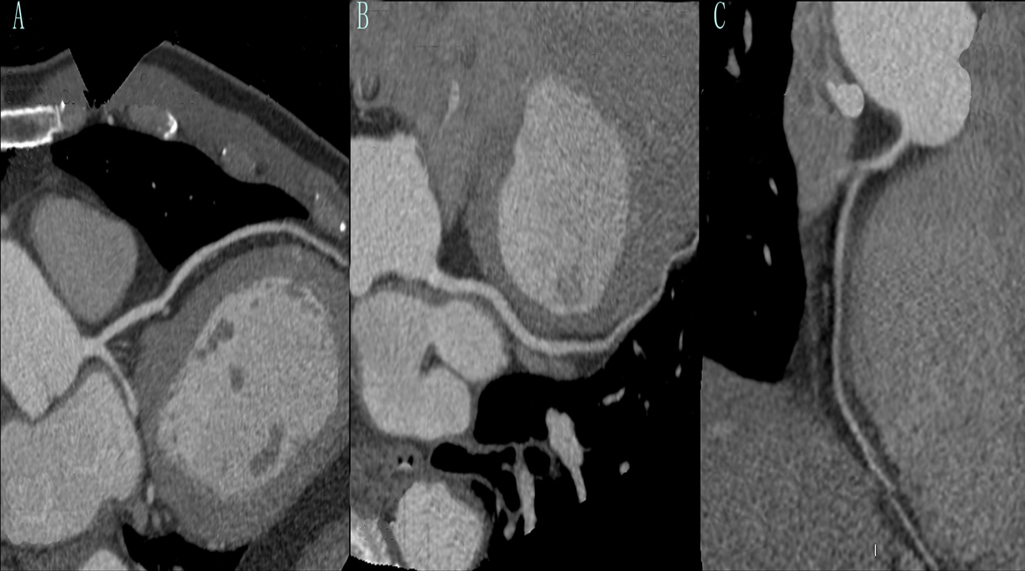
One example. A 54-year-old man with a history of chest pain underwent CT coronary angiography (curved MPR with 100kVp, protocol) and BMI of 26.4 kg/m^2^. We delivered the contrast medium (300 mgI/ml). A: left anterior descending; B: left circumflex; C: right main artery. The radiation dose was 2.45mSv. We can clearly make the diagnostic decision according to this figure: there is no calcified plaque or stenosis in left anterior descending, left circumflex and right main artery.
